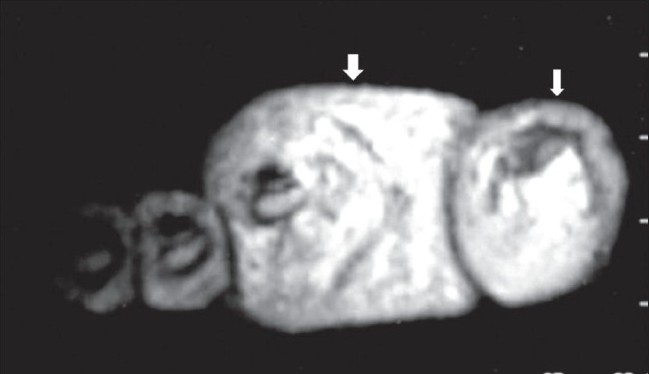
Case 2: Axial T2W MRI image shows fat-intensity soft tissue (arrow) surrounding these digits
